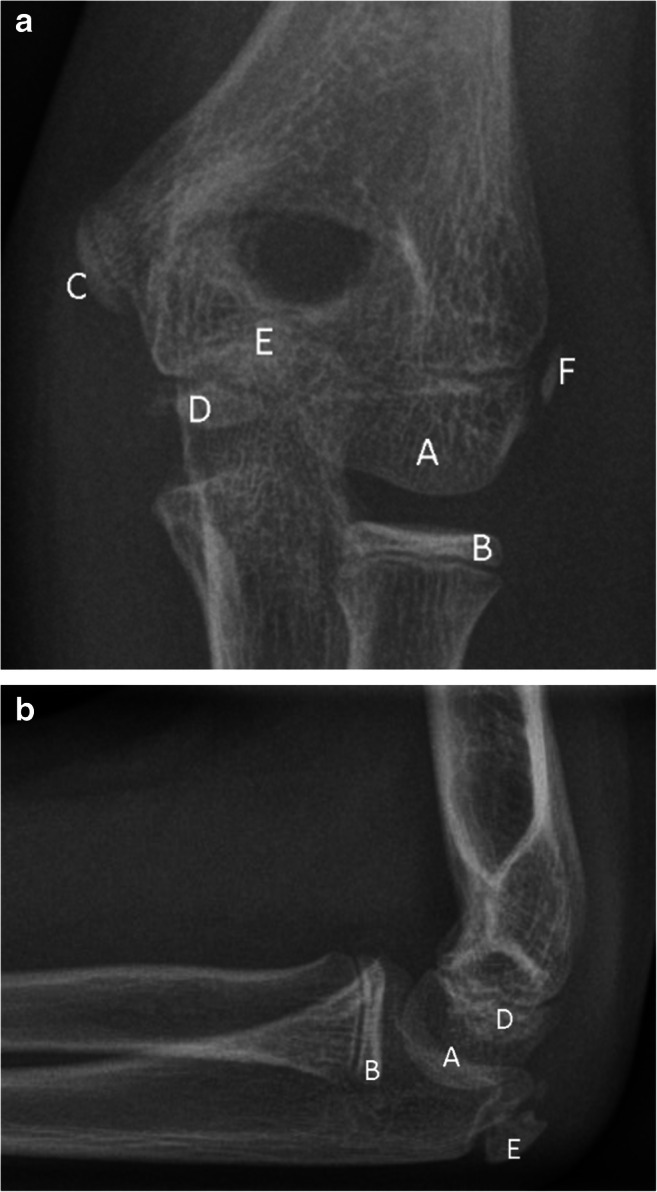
Normal radiograph of the left elbow of a 10-year-old girl in (a) anteroposterior projection and (b) lateral projection showing the six apophyses of the elbow joint: (A) capitellum, (B) radial head, (C) internal epicondyle, (D) trochlea, (E) olecranon, and (F) external epicondyle
